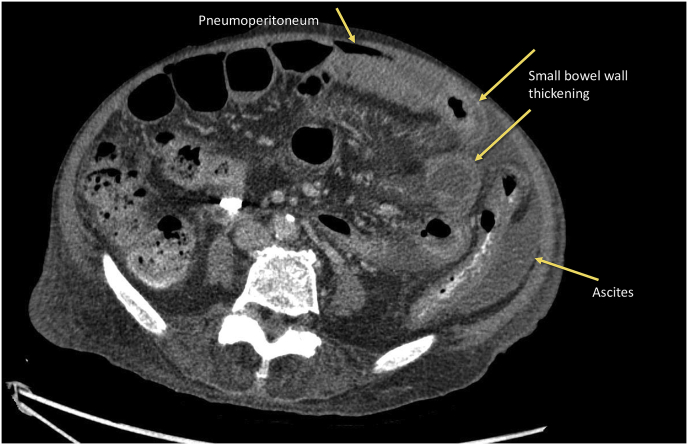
Abdominal CT scan demonstrated small pneumoperitoneum with air bubbles trapped in pelvic fluid.
